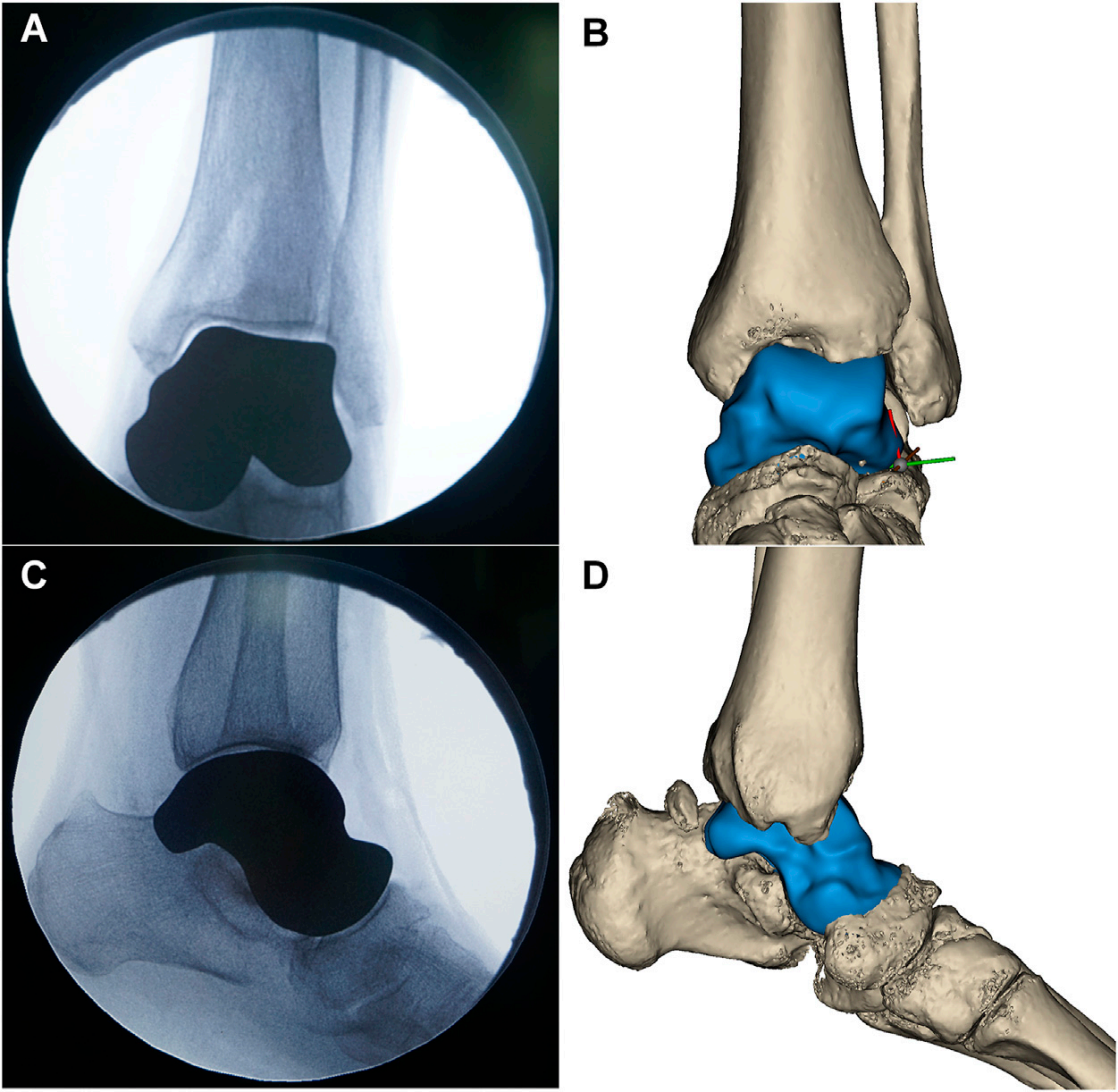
The position of the 3D printed talar prosthesis was confirmed by postoperative radiography (A,C), and matched with the preoperative 3D reconstructed results (B,D).
